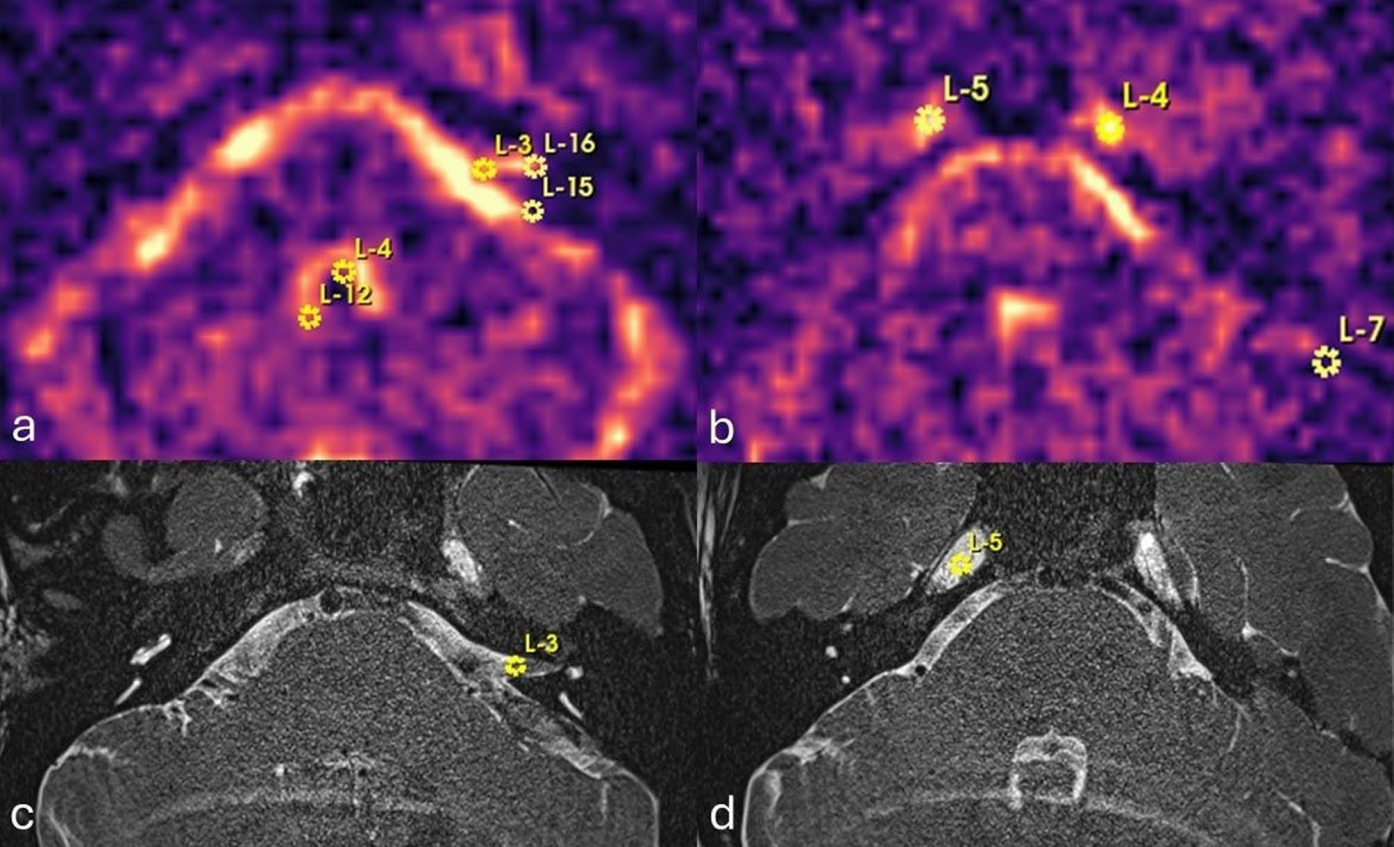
^23^Na MRI axial images demonstrating landmark placement for registration. These are placed in the (a) fourth ventricle (L-4, L-12), medial cerebello-pontine angle cistern (L-3, L-15) and internal auditory meatus (L-16) and (b) lateral cerebello-pontine angle cistern (L-7) and Meckel’s caves (L-4, L-5). Selected corresponding points are shown in (c) and (d) on the structural T2-SPACE sequences
